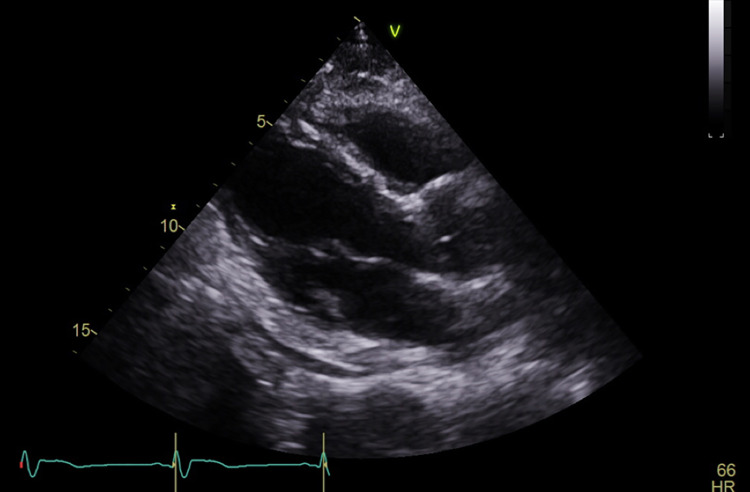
Echocardiogram with posterior pericardial effusion
